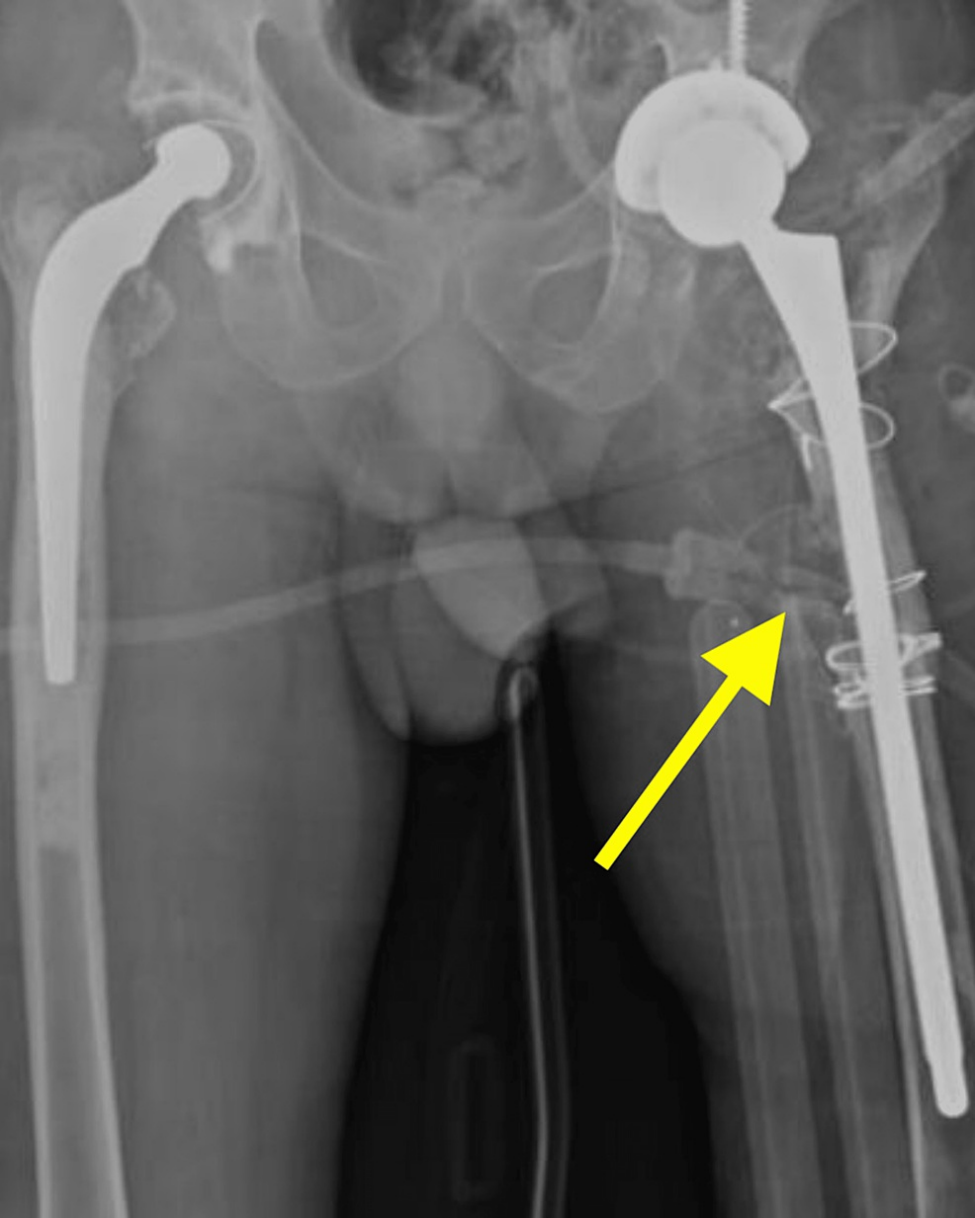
Post-operative radiograph of pelvis with both hip joints anteroposterior view shows periprosthetic femur fracture managed with revision total hip arthroplasty left side
